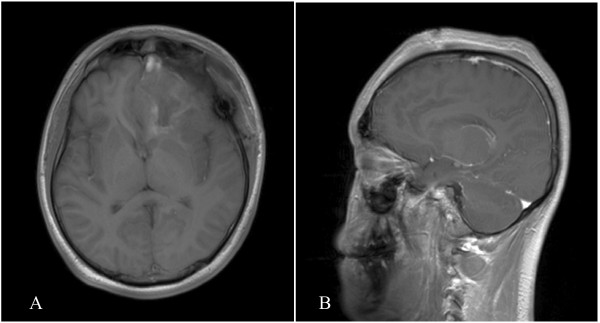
Follow-up MRI scan at six months. Axial (A) and coronal (B) MR images show no residual tumor tissues or tumor relapse in the left frontal lobe.
